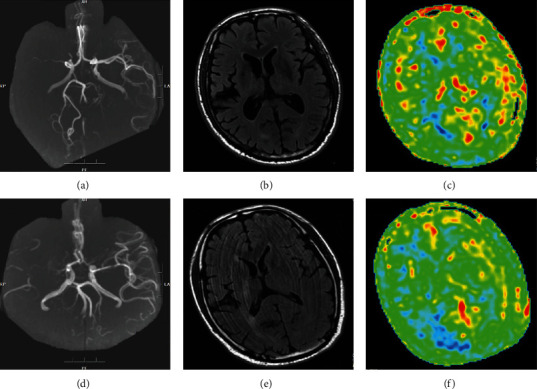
Regions of interest in the blood supply region of two patients' brains. (a) MRA indicated occlusion of M1 segment of the right MCA; (b) DWI showed negative; (c) ASL suggested that the CC was well established (PLD 2525 ms); (d) MRA showed that the M1 segment of the right MCA was occluded; (e) DWI showed negative; (f) ASL indicated insufficient collateral circulation (PLD 2525 ms).
